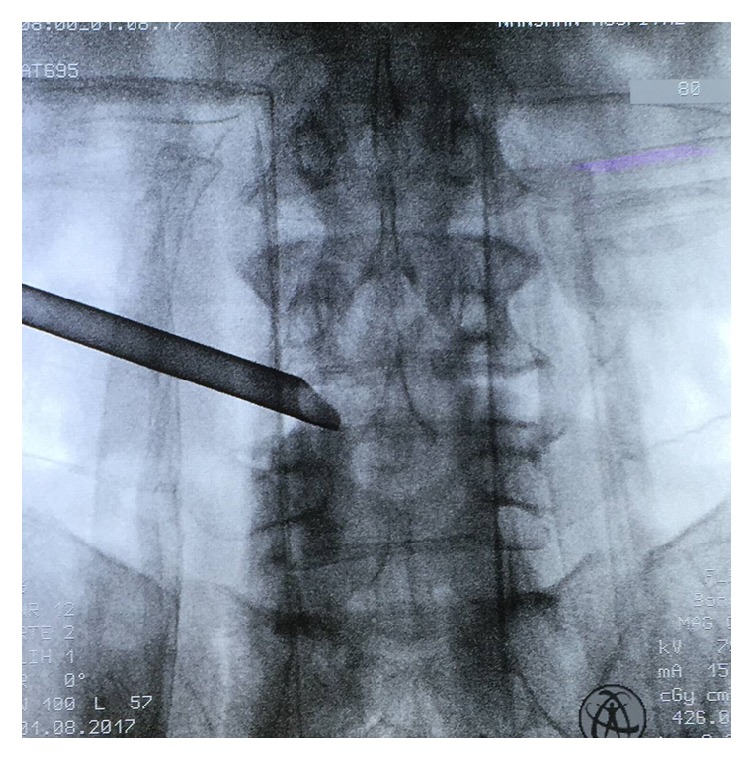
Radiography showing the working cannula has successfully reached the operation area at L4-5 level.
